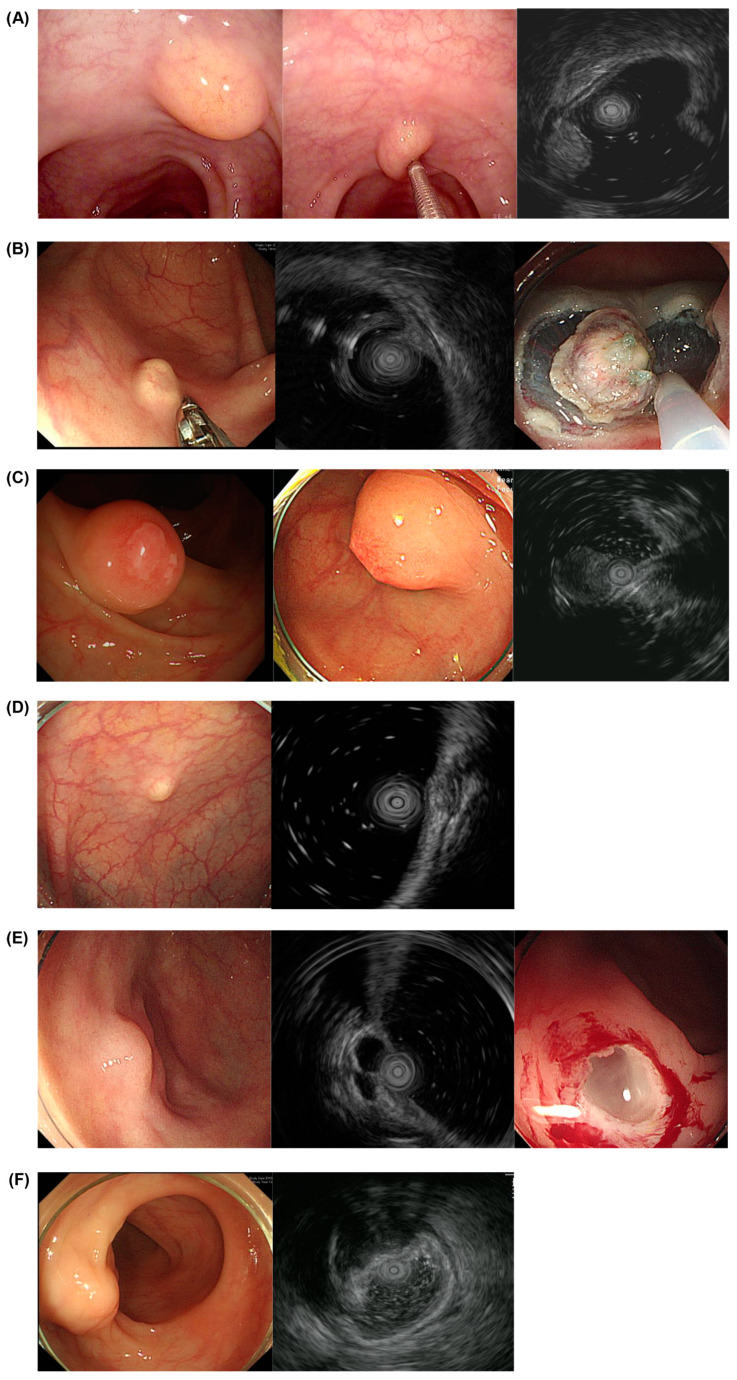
EUS findings of benign lesions. (A) Lipoma, (B) parasitic infection, (C) inflammatory fibroid polyp, (D) leiomyoma, (E) lymphangioma, and (F) endometriosis. EUS, endoscopic ultrasound.
